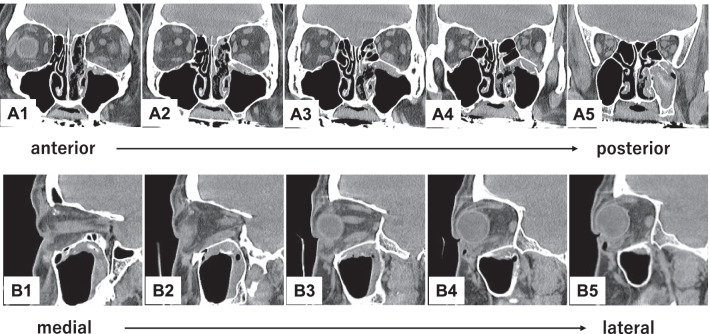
Postoperative computed tomography (CT) images. A1–5 are reformatted coronal CT images in anterior to posterior order. B1–5 are reformatted sagittal CT images in medial to lateral order. Images B3 and B4 show the implant inserted posterior to the inferior orbital fissure and in contact with the inferior margin of the greater wing of the sphenoid bone. The orbit and the maxillary sinus were completely separated by a bone–mucosal flap; the implant was not exposed to the maxillary sinus
